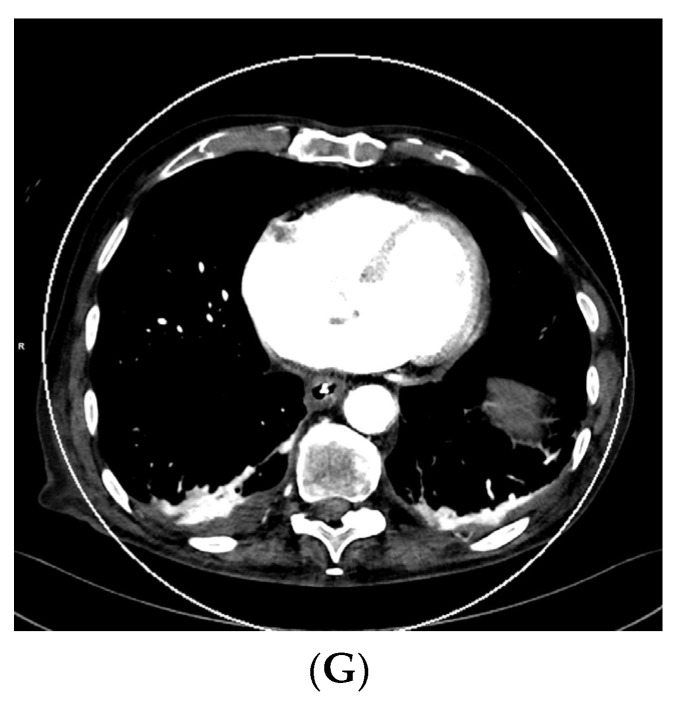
Acquisition of a DECT CTA of the thoracic aorta with a bad intravascular contrastation due to low output cardiac insufficiency in a 75-year-old male patient suffering from chest pain, tachycardia, and peripheral edema; (A) shows an axial view 120 kV VMI reconstruction of the descending aorta thoracalis with low intravascular contrast. Further, we see bilateral pleural effusions with adjacent partial atelectasis of the lung parenchyma. Aortic dissection could be ruled out as a cause for the clinical presentation; (B–G) show monoenergetic VMI reconstructions from 40 to 90 kV in 10 kV steps, with increasing contrast from the higher kV reconstruction of 90 kV (B) to the low-kV reconstructions with maximum intravasal contrast in the 40 kV reconstruction (G), which is closest to the K-edge of iodine, which is located at 33 kV. Accordingly, the K-edge peak is found in (G), as the highest intravasal attenuation is found here.
